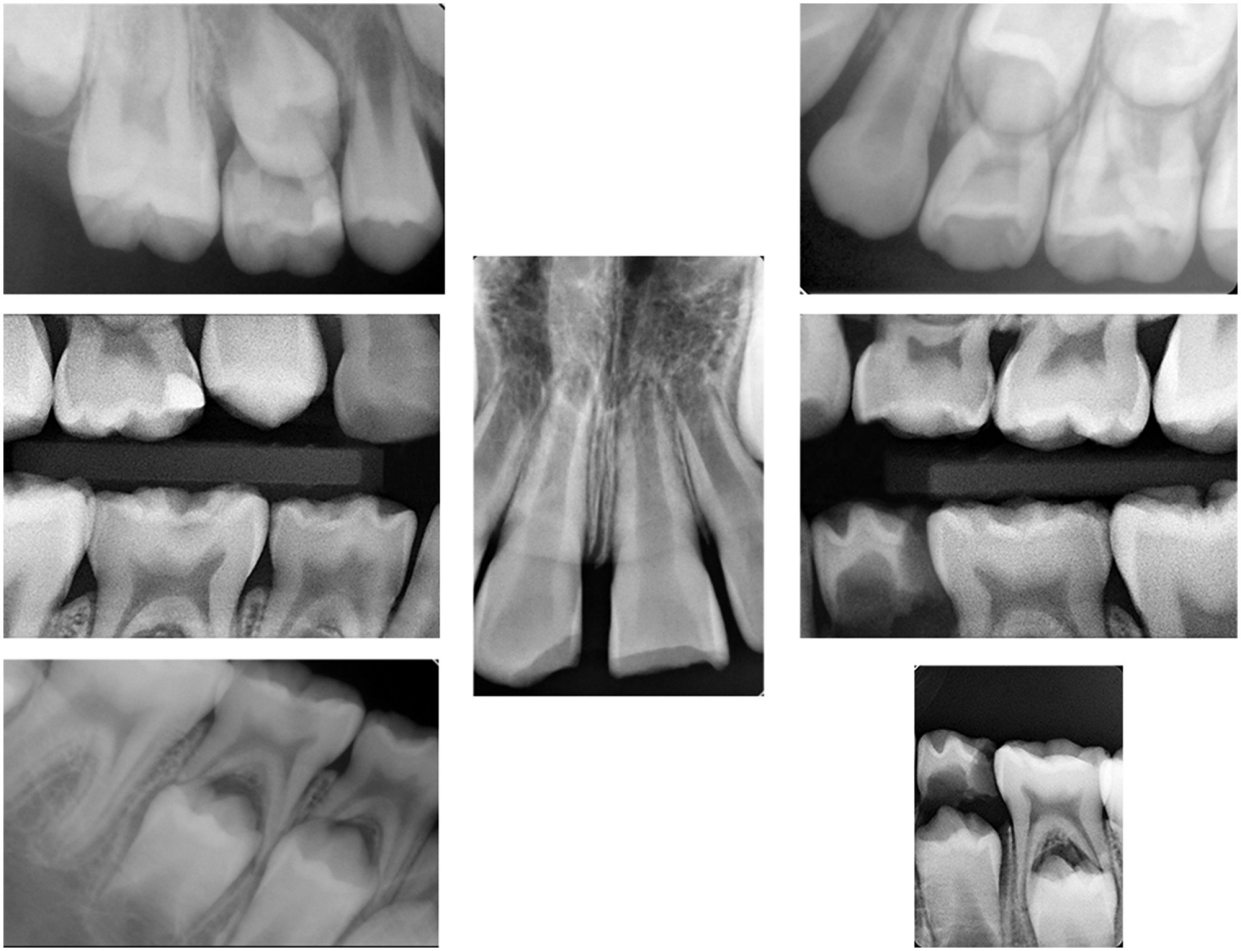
Preoperative intraoral radiographs.
